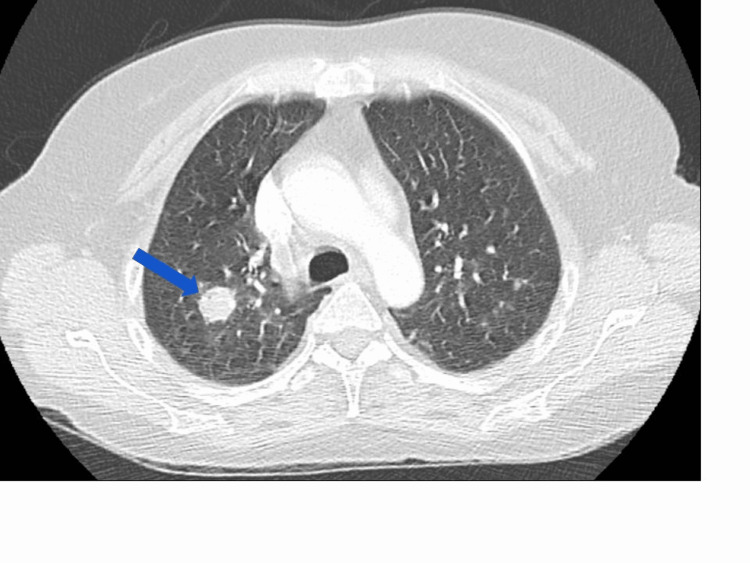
CT angiography of the chest showing the largest right lower lobe nodule (blue arrow).
